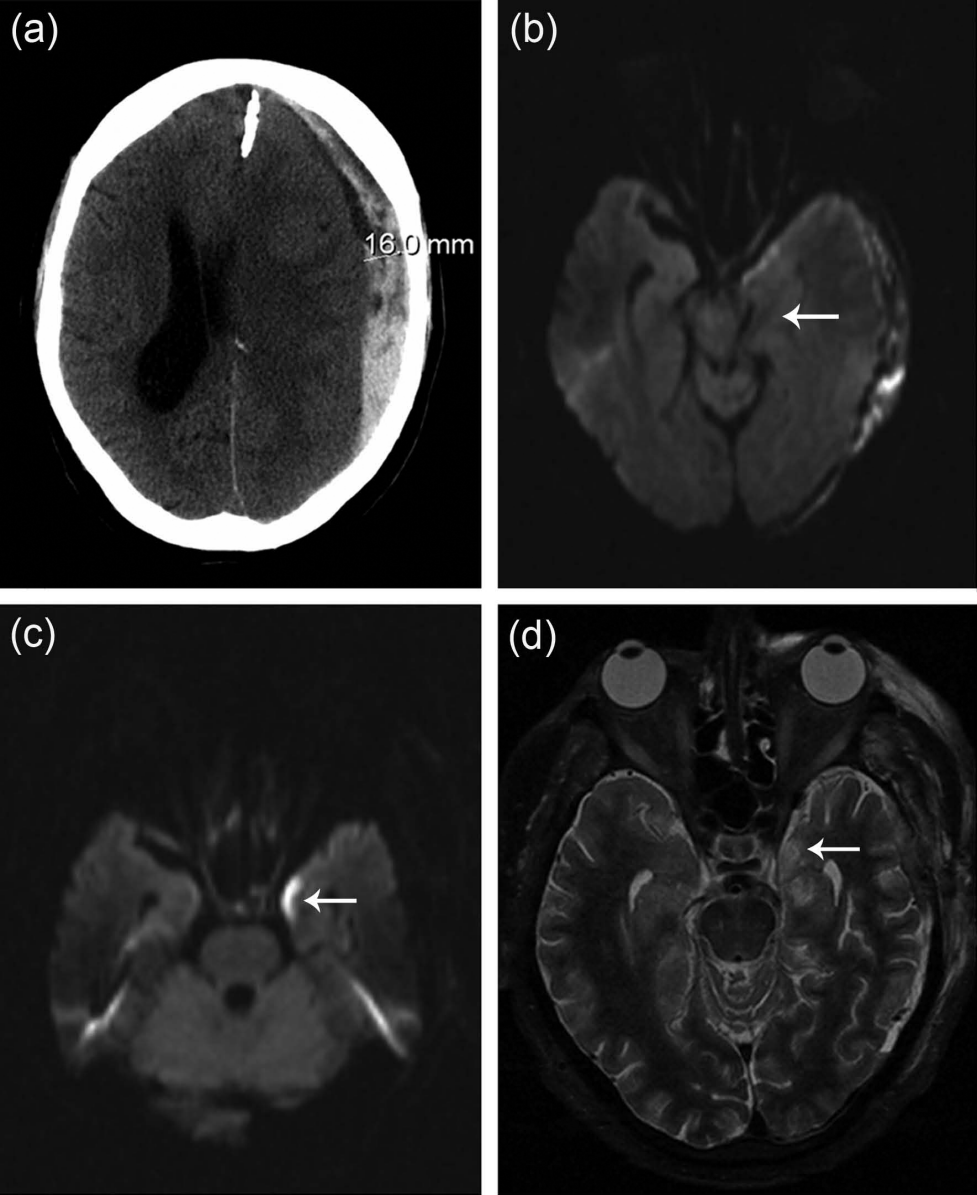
Computed tomography of subdural hematoma and cerebral magnetic resonance imaging depicting early uncal herniation and mesiotemporal ischemic brain injury. (A) Preoperative cranial computed tomography scan reveals space-occupying subdural hematoma covering the left convexity. The hematoma causes a considerable midline shift at the level of the lateral ventricles. (B) Early diffusion- weighted magnetic resonance imaging (diffusion-weighted imaging) depicts a slight uncal herniation and a midline deviation at the level of the chiasmatic basal cistern. (C) Diffusion-weighted imaging sequences four days after surgery show circumscribed signal hyperintensity in the left uncal region indicative of local ischemic injury. Note the close anatomic proximity to the distal prechiasmatic course of the left optic nerve. (D) Corresponding hyperintensity in T2-weighted images indicates edema of the left temporomesial lobe that is consecutive to hypoperfusion and residual to preceding uncal herniation.
